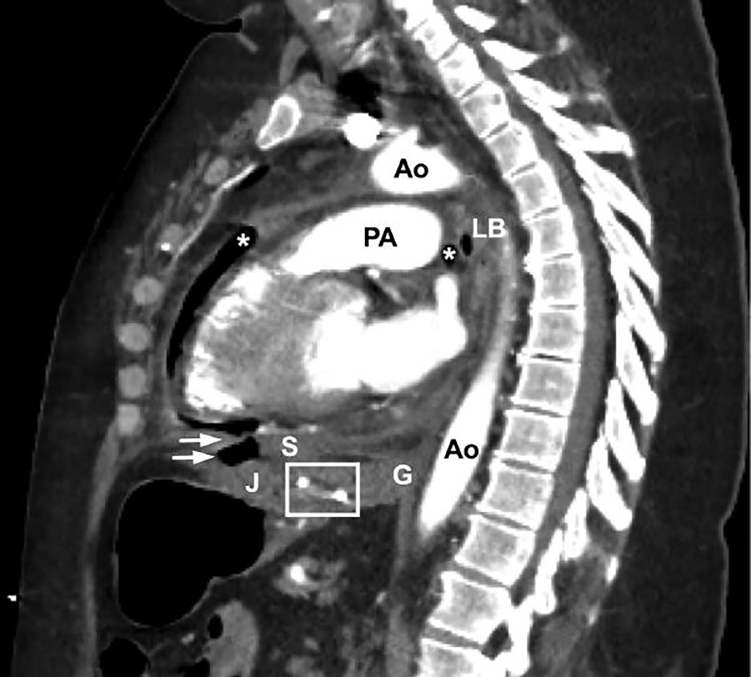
Computed tomography arteriography demonstrates pneumopericardium (*), the gastrojejunal anastomotic ring (box), and air adjacent to the inferior portion of the diaphragm, with notable disruption and thinning of the diaphragmatic contour (arrows). *Ao*, aorta; *G*, gastroesophageal junction; *J*, jejunum; *LB*, left mainstem bronchus; *PA*, pulmonary artery; *S*, stomach.
